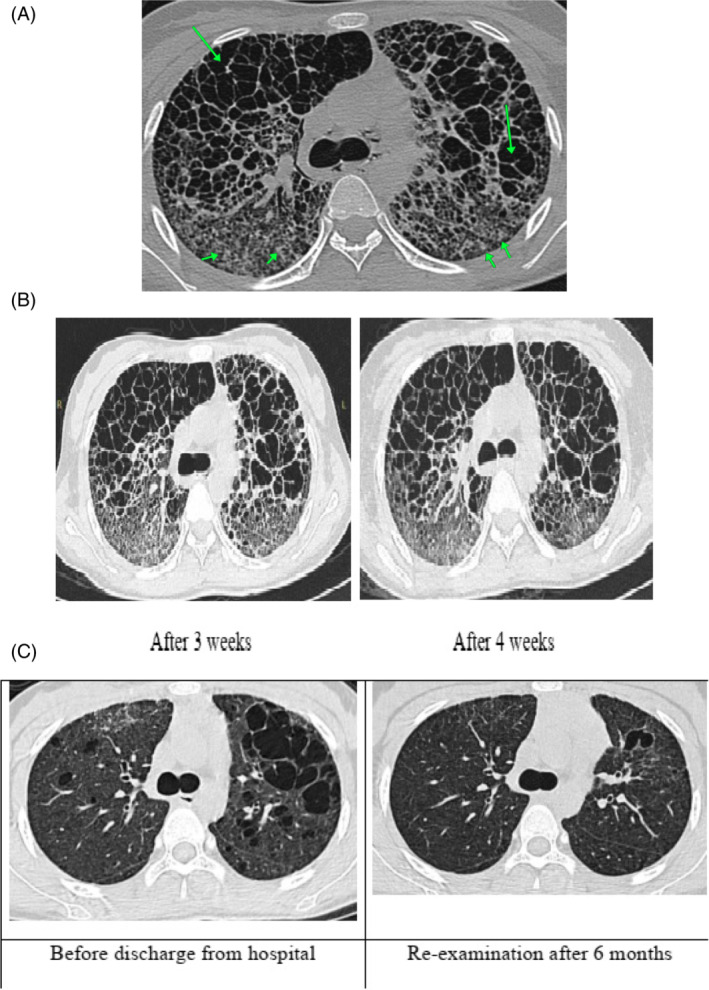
(A) Chest HRCT taken 1 week after initial treatment. Cystic lesion of upper lobes was markedly increased in size with arrangement suggestive of panacinar emphysema (green arrows, with arrowhead point to centrilobular vessel). Several clustered micronodules and microcystic lesions are seen in the hyperdensity regions of the lower lobes. (B) HRCT films were taken 3 and 4 weeks after initial treatment. The cystic lesion of both upper lobes continued to increase in size, some of which fused together to become bullous emphysema. Cystic lesions of lower lobes also increased in number and size. (C) HRCT films taken before discharge and at re‐examination after discharged 6 months. Most of the bullous has been resolved and lung parenchyma restored to normal.
